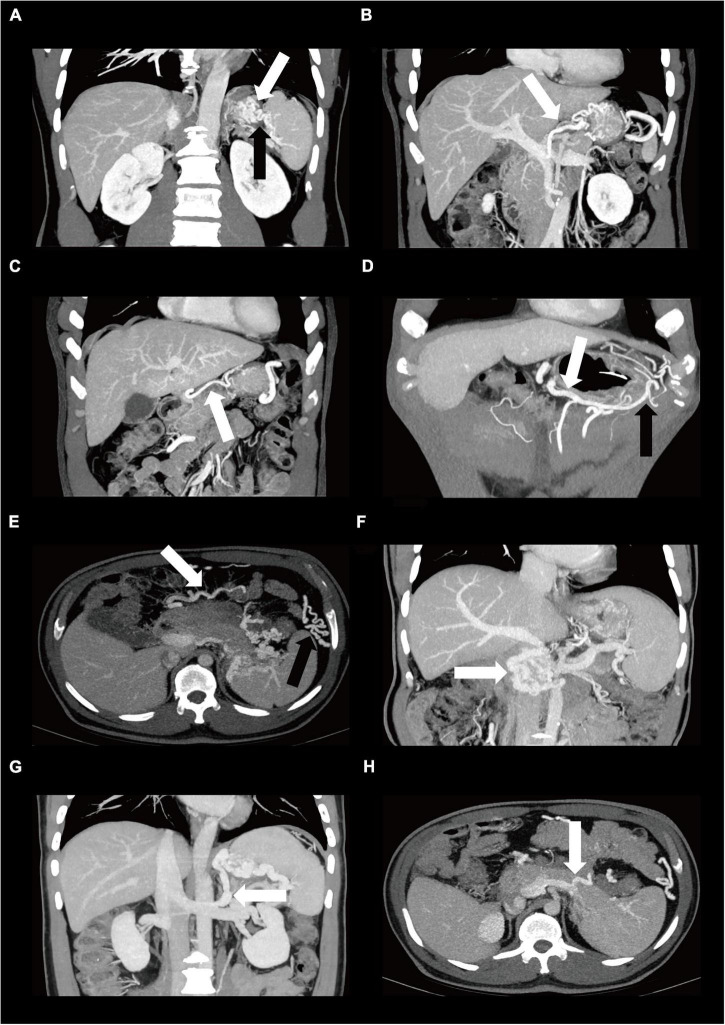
The Contrast-enhanced CT images of varices in patients diagnosed as SPH. One of the main collateral pathway is through enlarged short gastric varices (A, black arrow) and gastric fundus varices (A, white arrow) *via* gastric coronary varices (B, white arrow) or rarely *via* right gastric varices (C, white arrow) drained into the portal vein. Another collateral pathway is through left gastroepiploic varices (D, black arrow) *via* right gastroepiploic varices (D, white arrow) drained into the superior mesenteric vein. Other abnormal varices due to portal hypertension include middle colonic vein (E, white arrow), omentum branch of the gastroepiploic vein (E, black arrow), pancreaticoduodenal vein (F, white arrow), left adrenal vein (G, white arrow), and dorsal pancreatic vein (H, white arrow). CT, computed tomography, SPH, sinistral portal hypertension.
